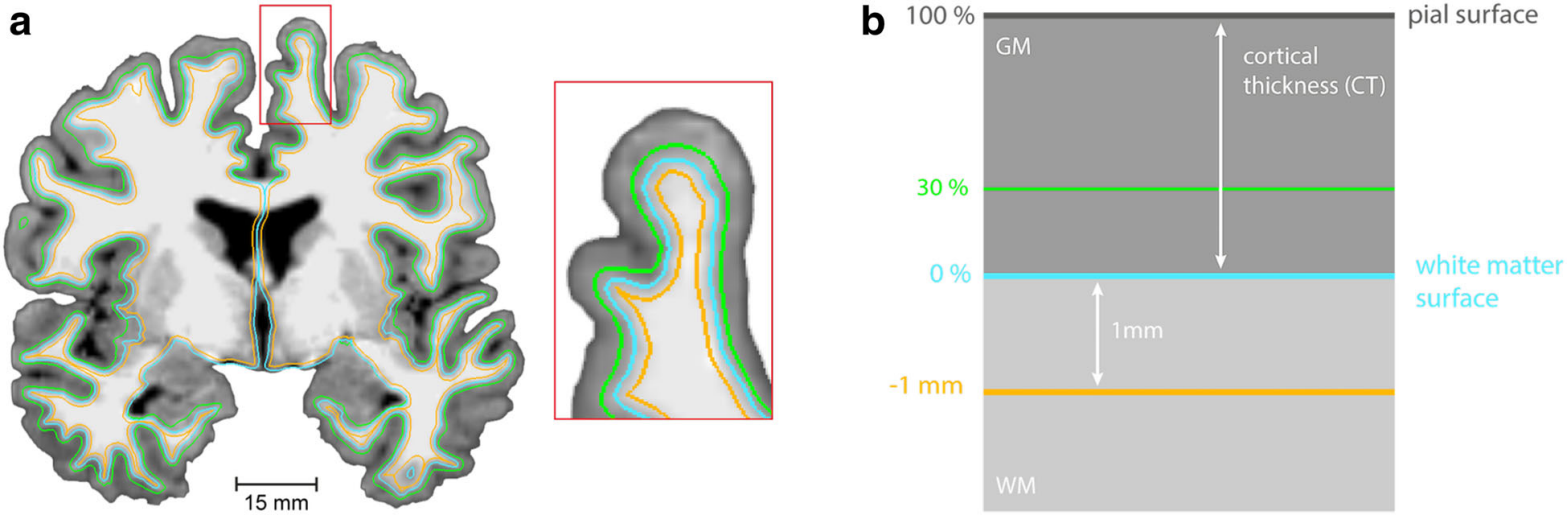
a Grey and white matter signal intensity sampling procedure. b At each cerebral vertex, GWC was calculated as the percentage of grey matter intensity (GMI) sampled at 30% cortical thickness (CT) relative to the white matter intensity (WMI) at 1 mm below the grey-white matter boundary. *Note. GM* grey matter, *WM* white matter
